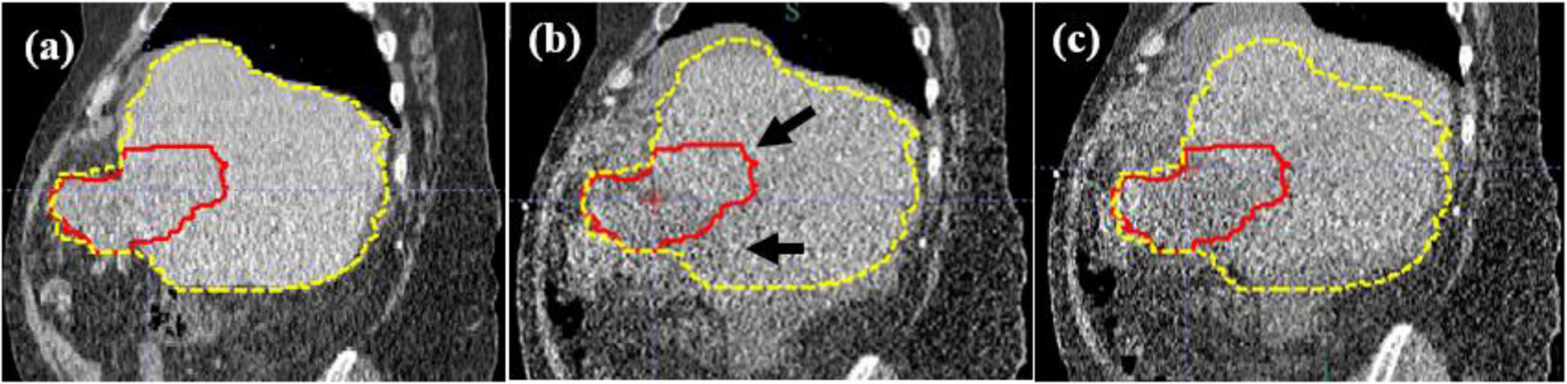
Computed tomography (CT) images showing the maximum manual shift correction of 24.3 mm. a Planning CT showing the contours of the liver (dotted yellow) and the gross tumor volume (GTV; solid red). b Result of automatic alignment, with the contours of the liver and GTV overlaid on top of the daily CT. The black arrows show the mismatch in the contour and the actual location of the GTV. c Result of manual shift correction
